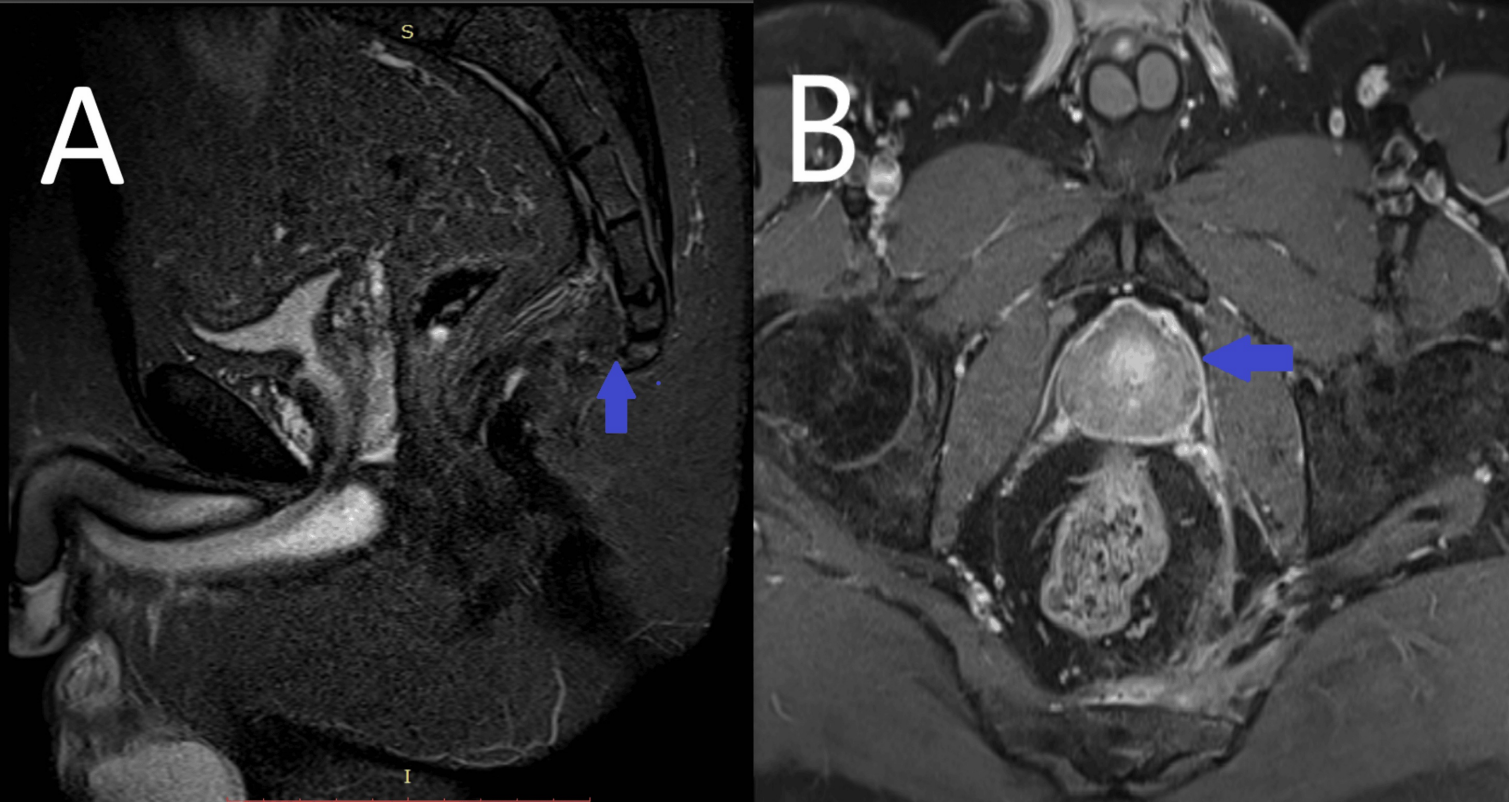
Postoperative MRI images A: After treatment, on the short tau inversion recovery (STIR) sagittal image, the hyperintensity in the vertebrae disappeared, and the inflammatory soft tissue changes in the presacral area significantly decreased. B: On the post-contrast T1 fat-saturated transverse image, pathological enhancement in the vertebrae disappeared, and the abscess pouch was no longer present.
